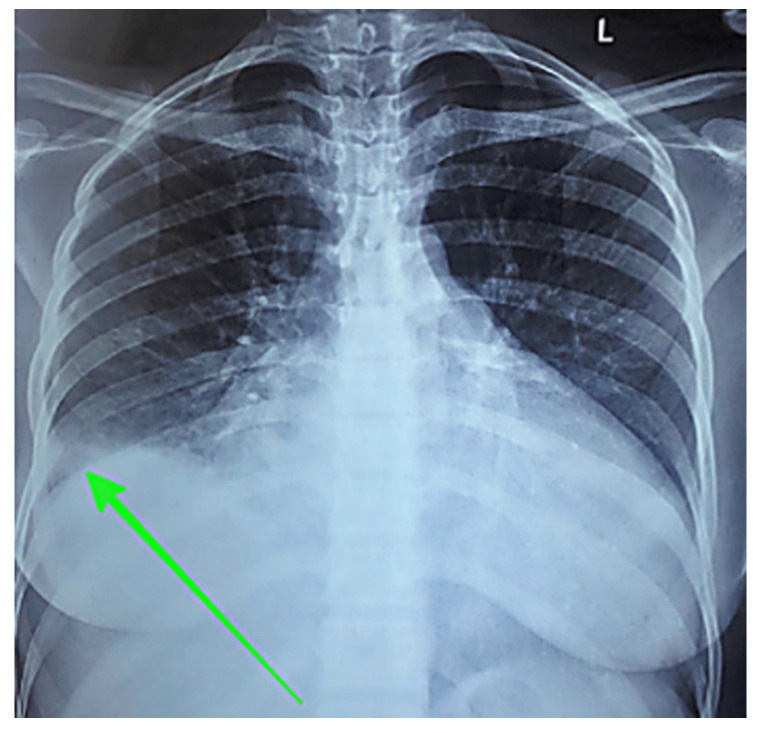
Representative chest X-ray example from the test set with green arrow indicating a right-sided pleural effusion.
